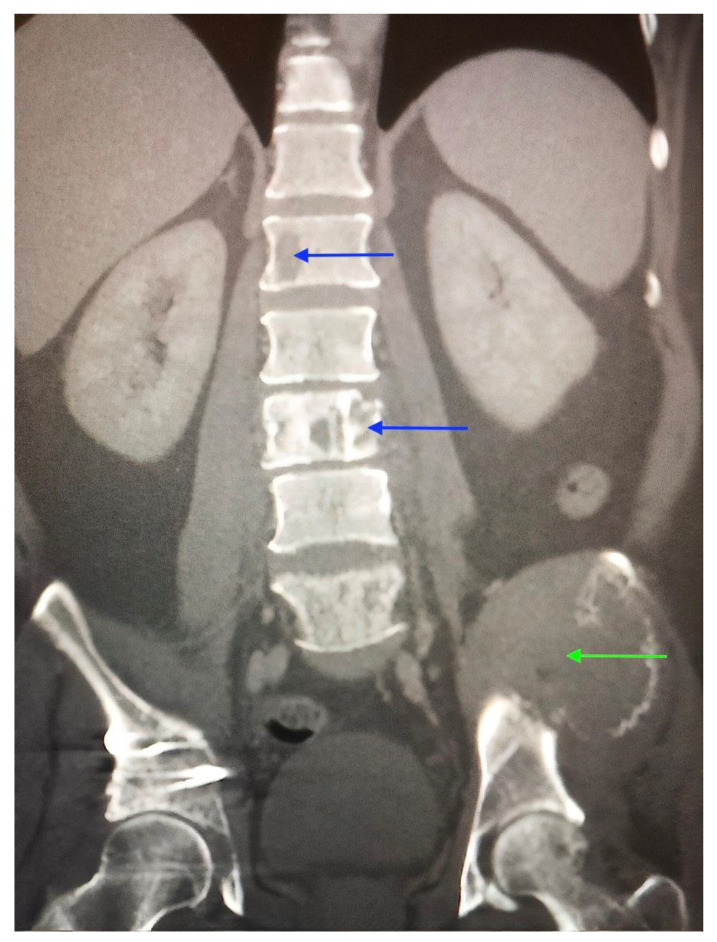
CT scan of the spine and pelvis highlighting multiple areas of bony destruction in the thoracic and lumbar vertebrae. (blue arrows) There is a large mass in the left iliac bone. (green arrow).
